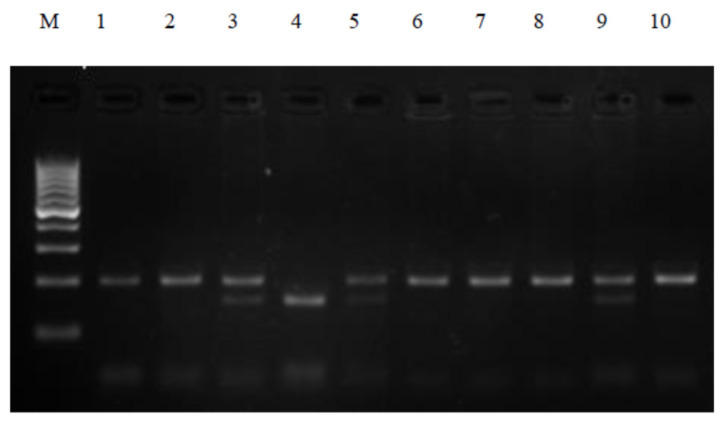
Restriction analysis of the 609C > T polymorphism in the NQO1 gene. Lane M—100 bp ladder marker; lane 1,2,6–8,10—CC genotype; lane 3,5,9—CT genotype; lane 4—TT genotype.
